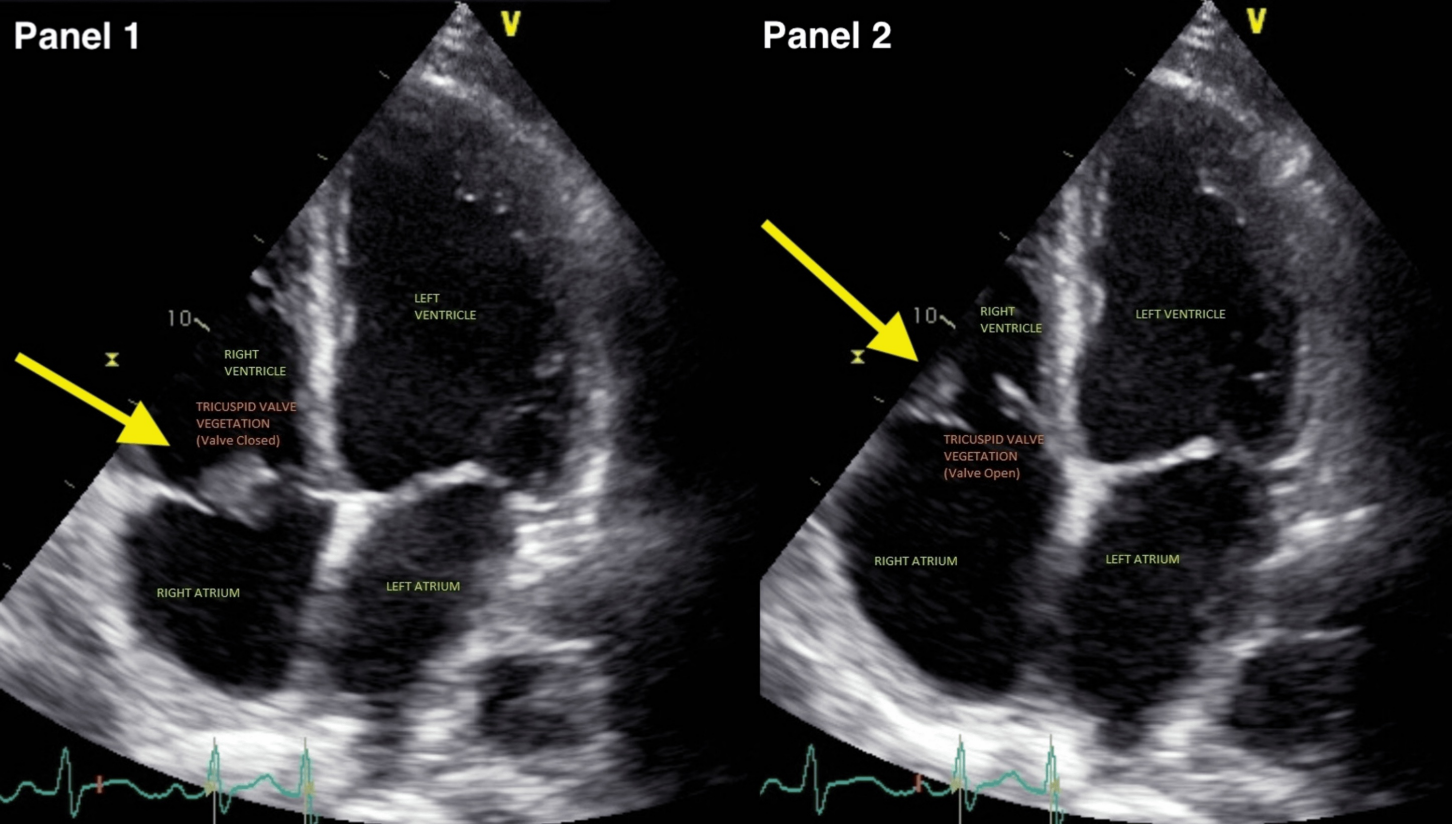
Echocardiogram showing large tricuspid valve vegetation in a patient with Streptococcus thoraltensis endocarditis. This figure contains two panels. Panel 1 shows the echocardiogram with the tricuspid valve vegetation visible when the valve is closed. Panel 2 shows the vegetation with the valve open, highlighting the dynamic nature of the vegetation on the tricuspid valve.
